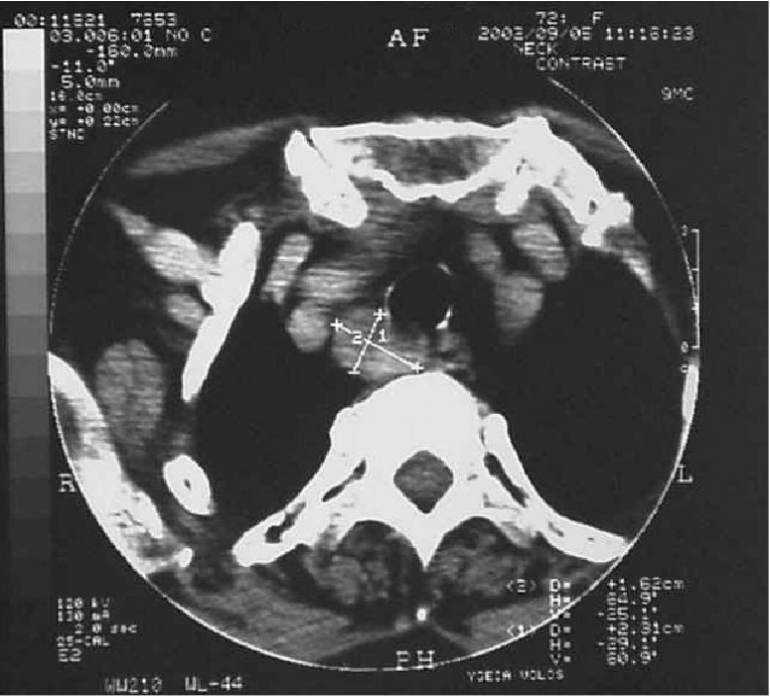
CT scan showing a paraesophageal, retrotracheal mass, close to the thoracic inlet
